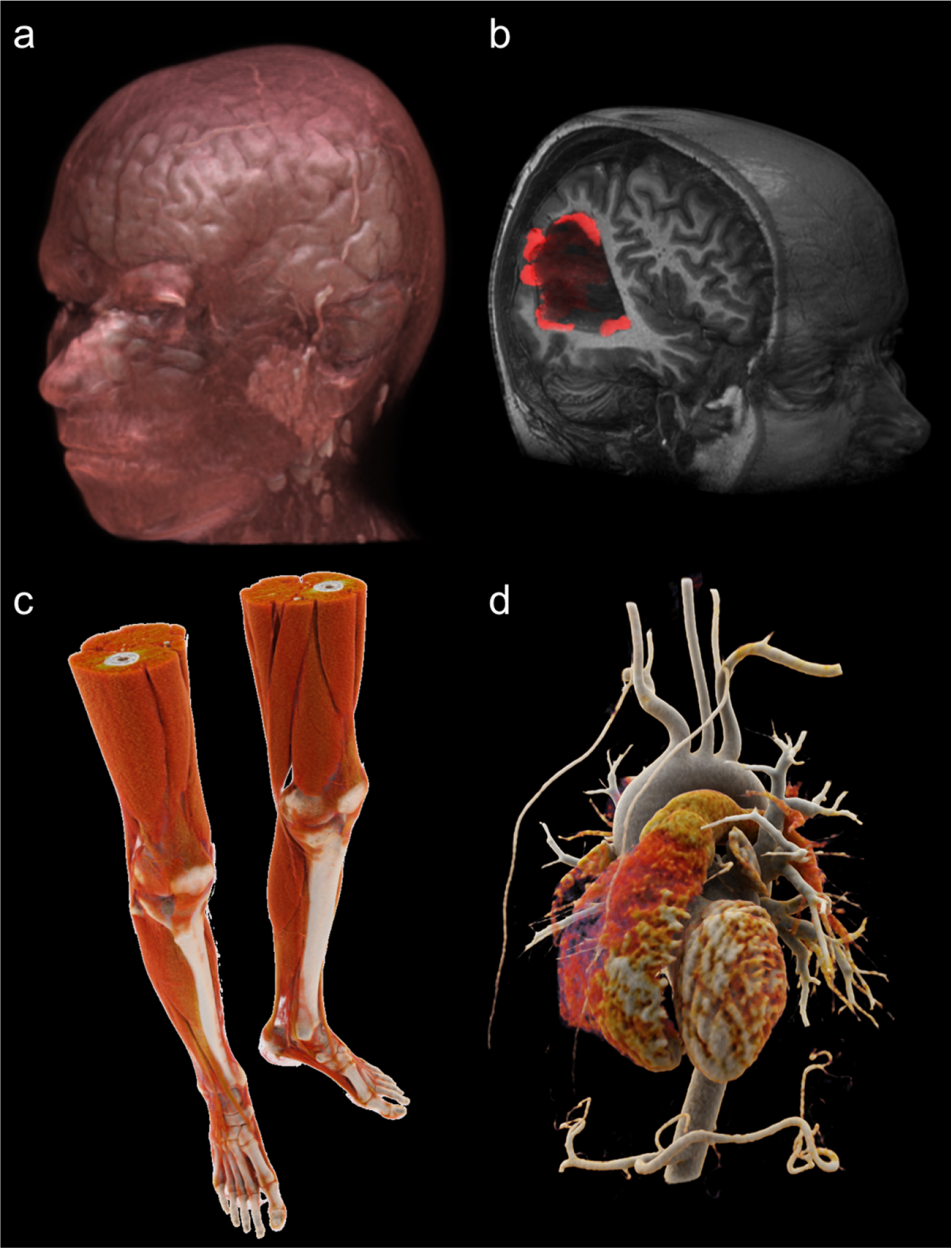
Current possibility for 3D volume rendering (VR). VR post-processed from MRI acquisition (a, b). Hyper-realistic cinematic VR processed from CT scan acquisition (c, d)
